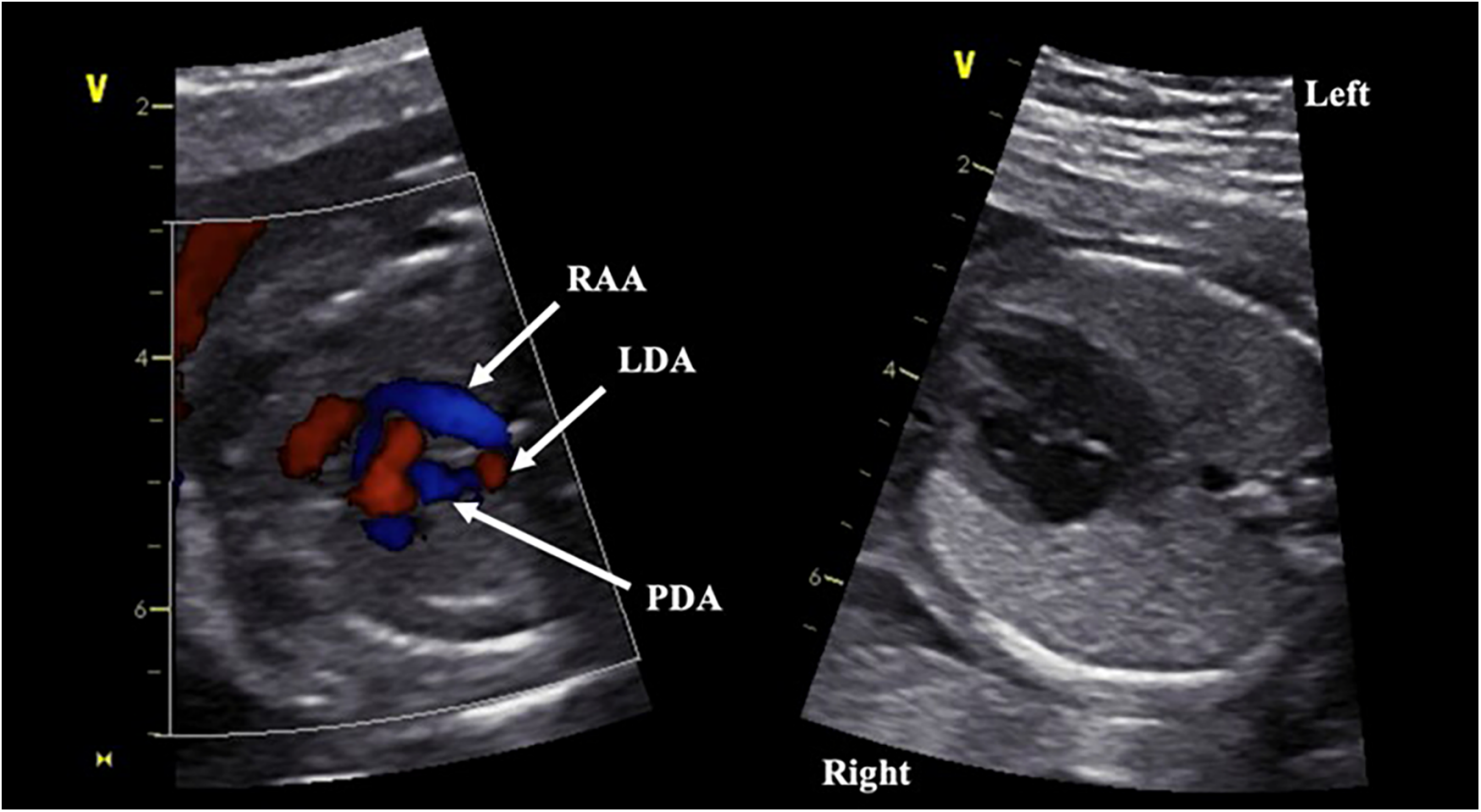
Fetal echocardiographic images at 20 weeks gestation. Panel on the left demonstrates vascular ring formed by the right circumflex aortic arch with left patent ductus arteriosus. Panel on the right demonstrates left sided descending aorta. RAA, right aortic arch; LDA, left descending aorta; PDA, patent ductus arteriosus.
